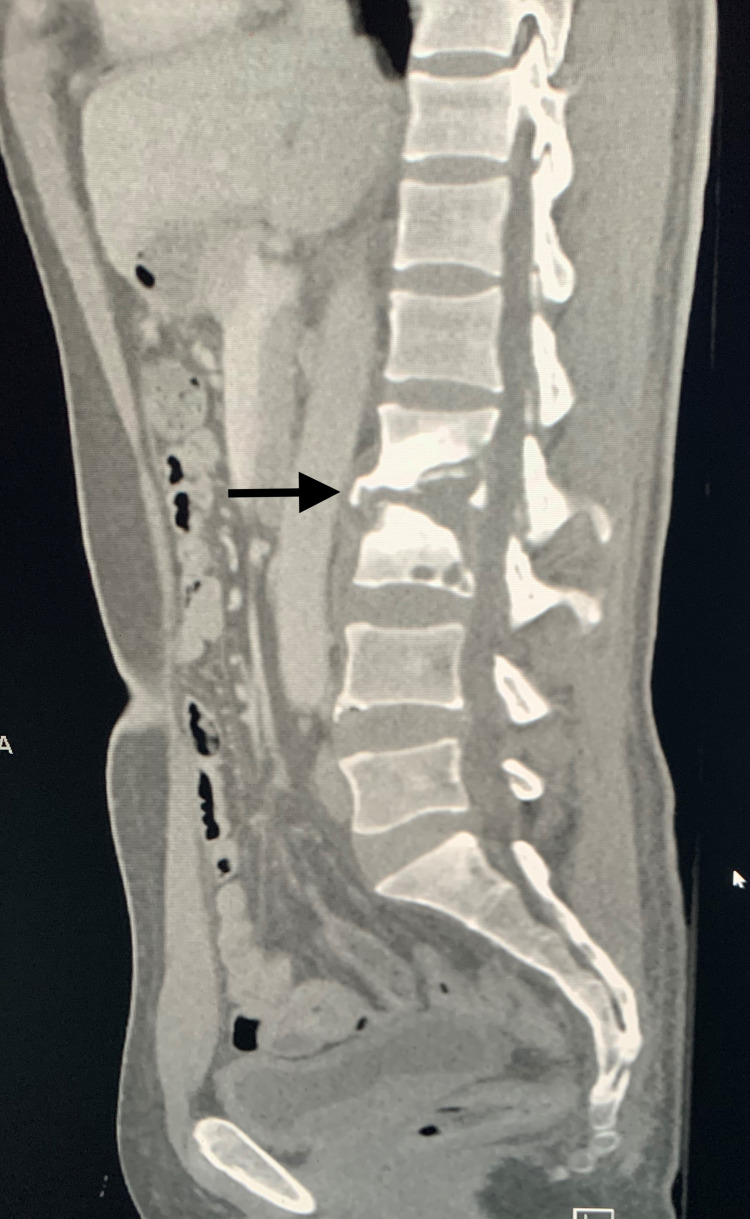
Sagittal CT of the abdomen at the level of the lumbar spine Sagittal image of the abdomen at the level of the mid lumbar spine utilizing bone windows. This demonstrates findings of chronic discitis/osteomyelitis at the L2/L3 level with evidence of a small prevertebral fluid collection.
